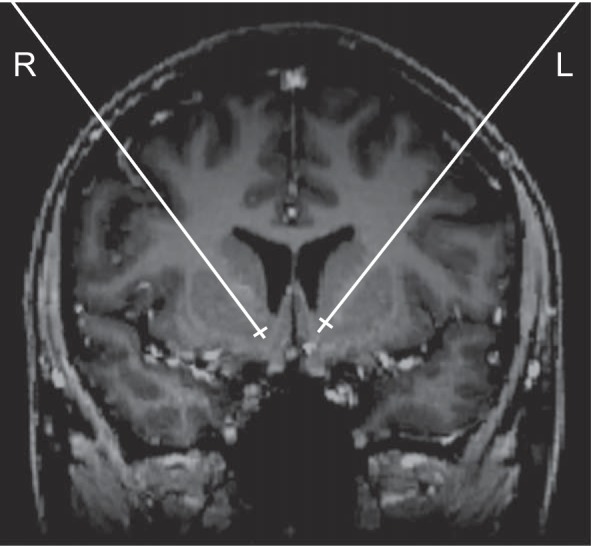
Exemplary presurgical MRI in 1 patient (*P6*) showing a projection of the planned placement of electrodes in bilateral NAcc onto a coronal MRI slice through the striatum.
